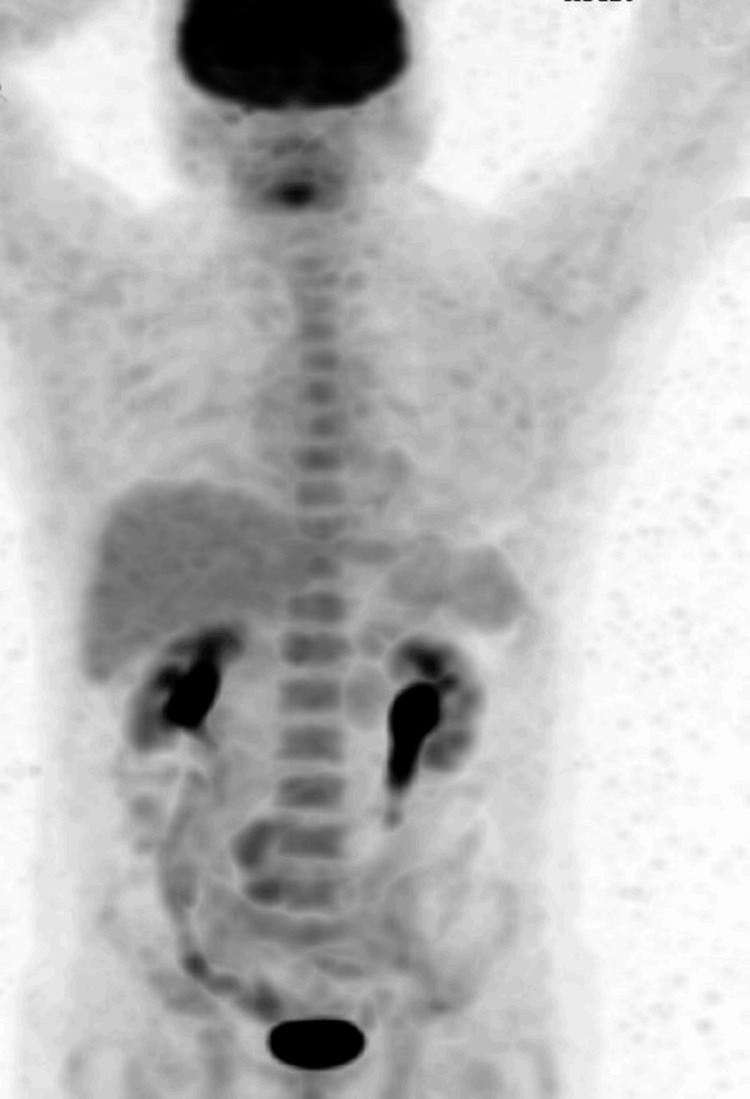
18-FDG PET scan after 11 weeks of therapy was negative for oncological disease (complete response) 18-FDG: fludeoxyglucose F18; PET: positron emission tomography
